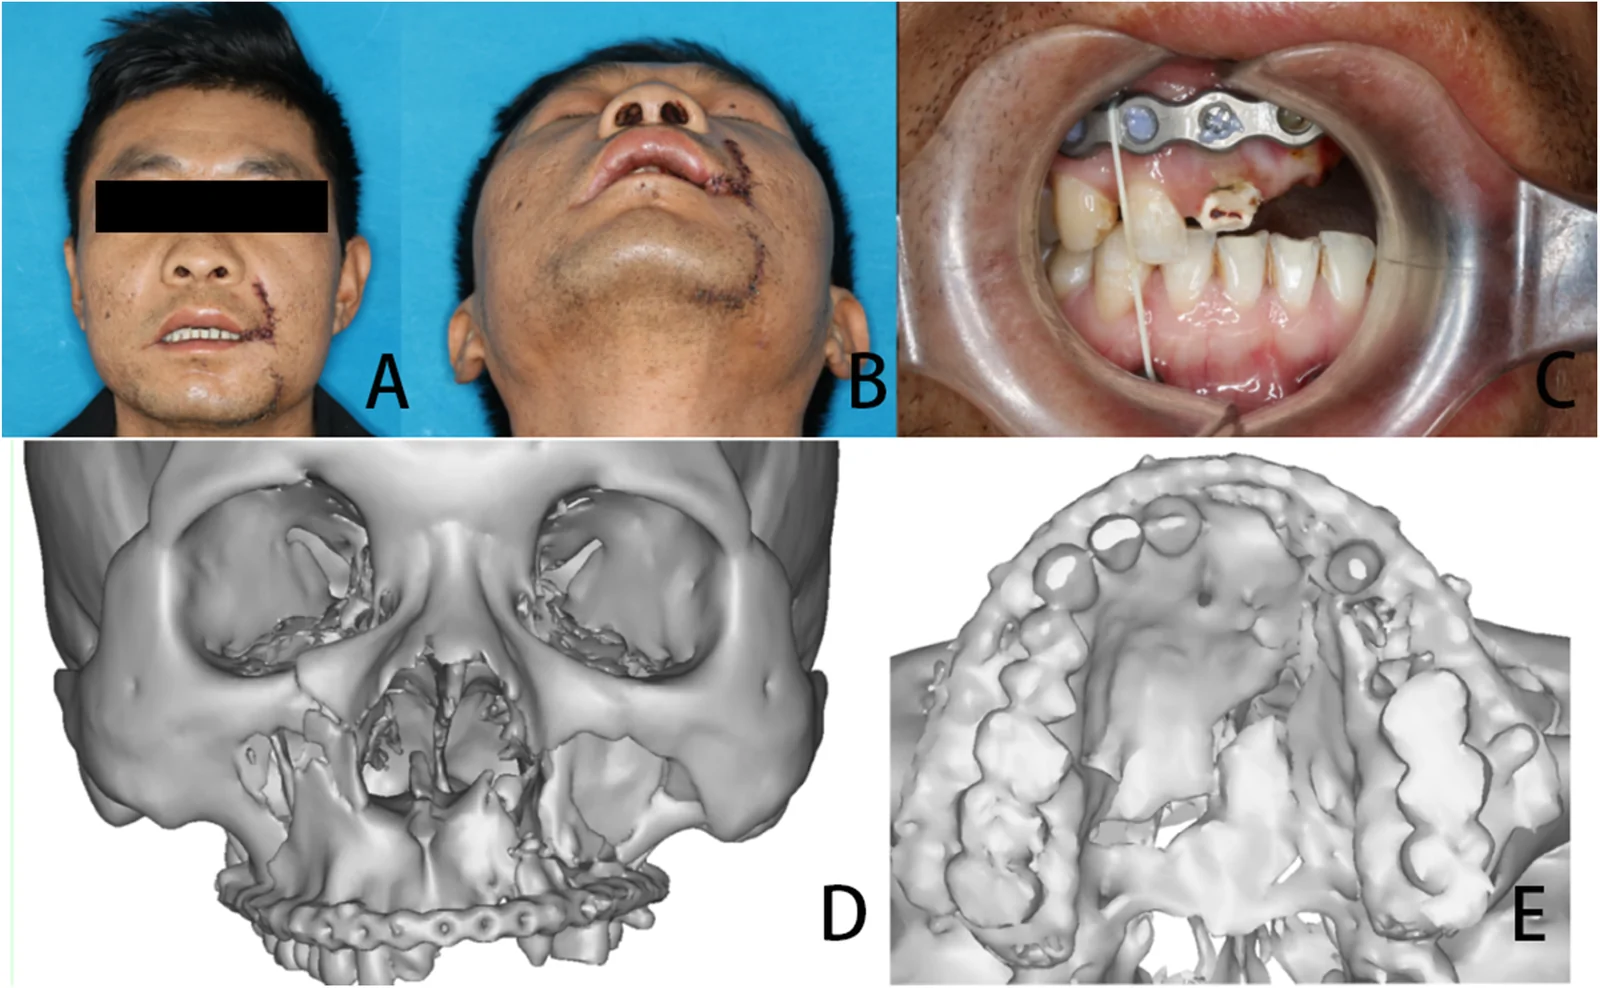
Postoperative clinical and radiographic images of case 1. (A,B) Extraoral photographs. (C) Intraoral photograph. (D,E) Three-dimensional CT reconstructions.
